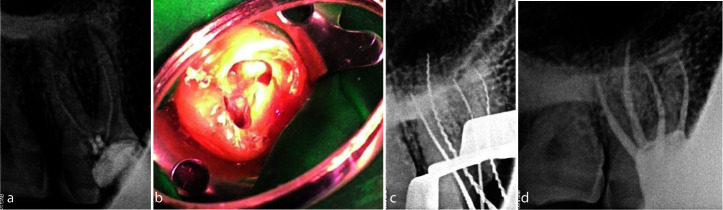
a: Pre-operative intraoral periapical radiograph, b: Access opening showing two palatal canal orifices, c: Working length radiograph, d: Post obturation intraoral periapical radiograph of tooth #16
